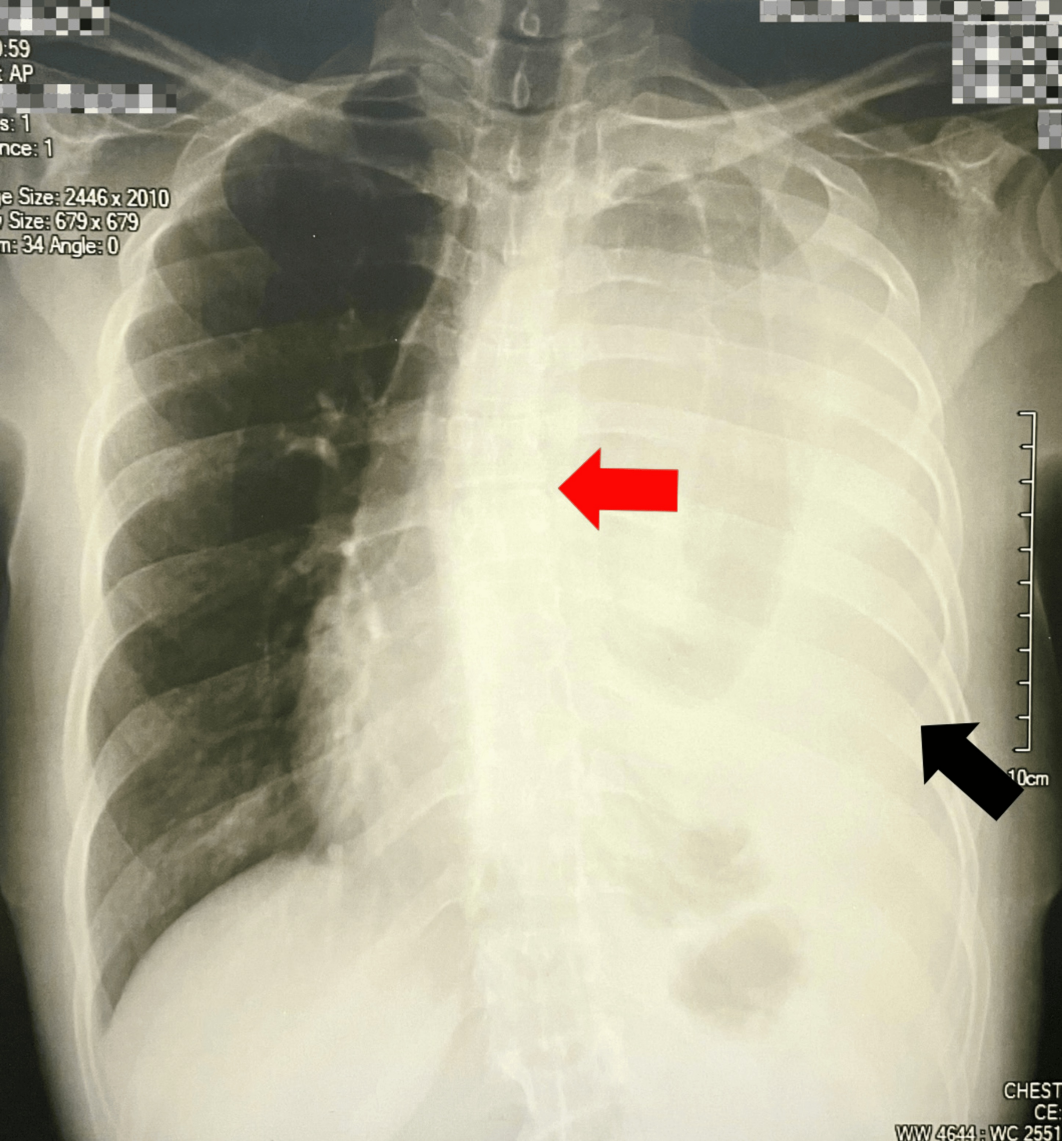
Chest X-ray The red arrow indicates the displacement of the structures towards the right hemithorax. The part indicated by the black arrow suggests what has been described as "massive pleural effusion".
